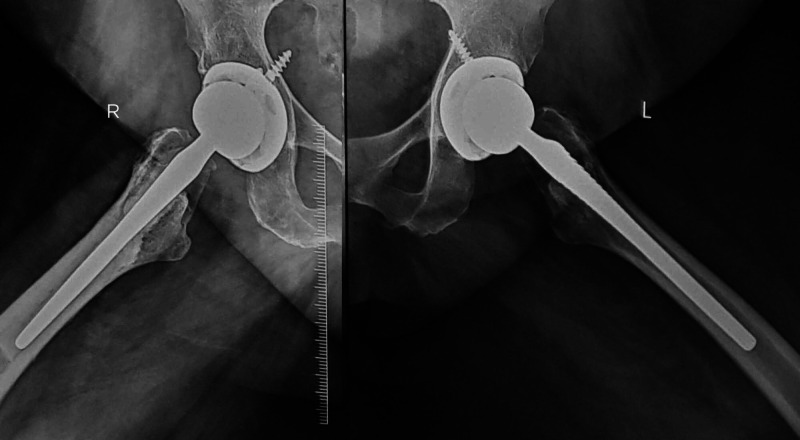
Lateral view both hips radiograph at two years follow up showed no component loosening or failure.
